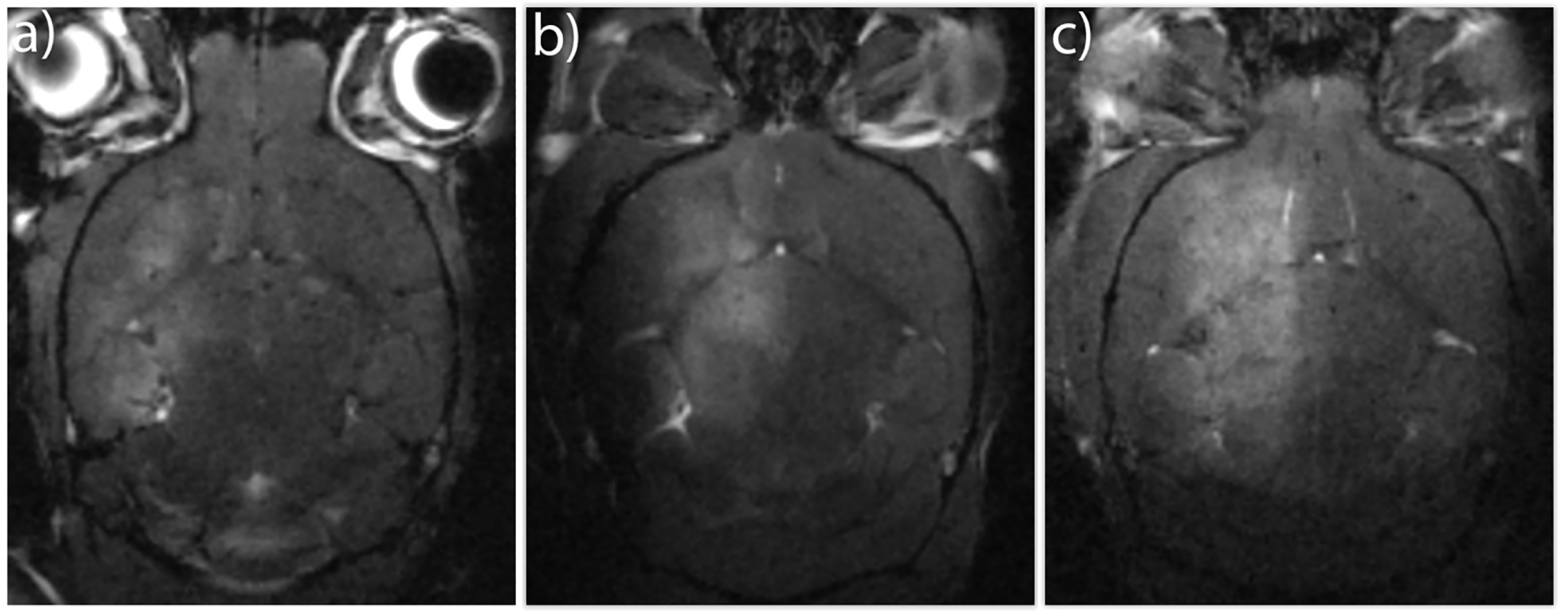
FUS-mediated BBB opening with PEBCA NP-MBs. Different extents of BBB opening are shown in panels a-c). The three brains show increasing amount of gadolinium-based contrast in the treated region, from hardly any contrast a), to large areas of high intensity contrast c) compared to the non-treated contralateral hemisphere. MRI images were normalized so that areas not exposed to FUS in different images have a comparable intensity. FUS parameters: 1.1 MHz, 5 min, pulse repetition frequency (PRF) 0.33 Hz, estimated *in situ* pressure 0.31–0.34 MPa, 10 ms burst length.
